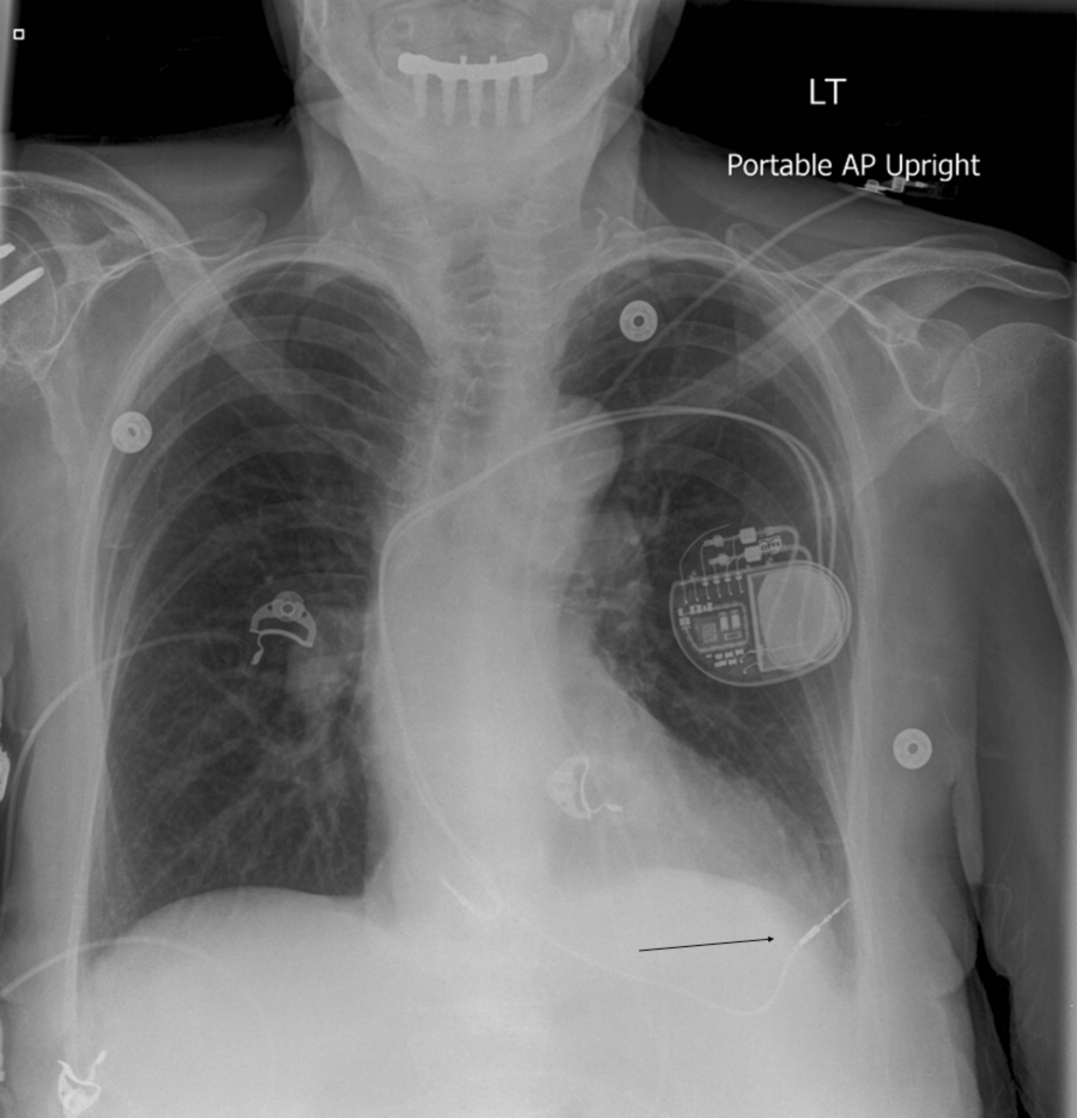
Chest X-ray of displaced lead This image shows a displaced pacemaker lead which has migrated through the myometrium and pericardium into the chest well.
